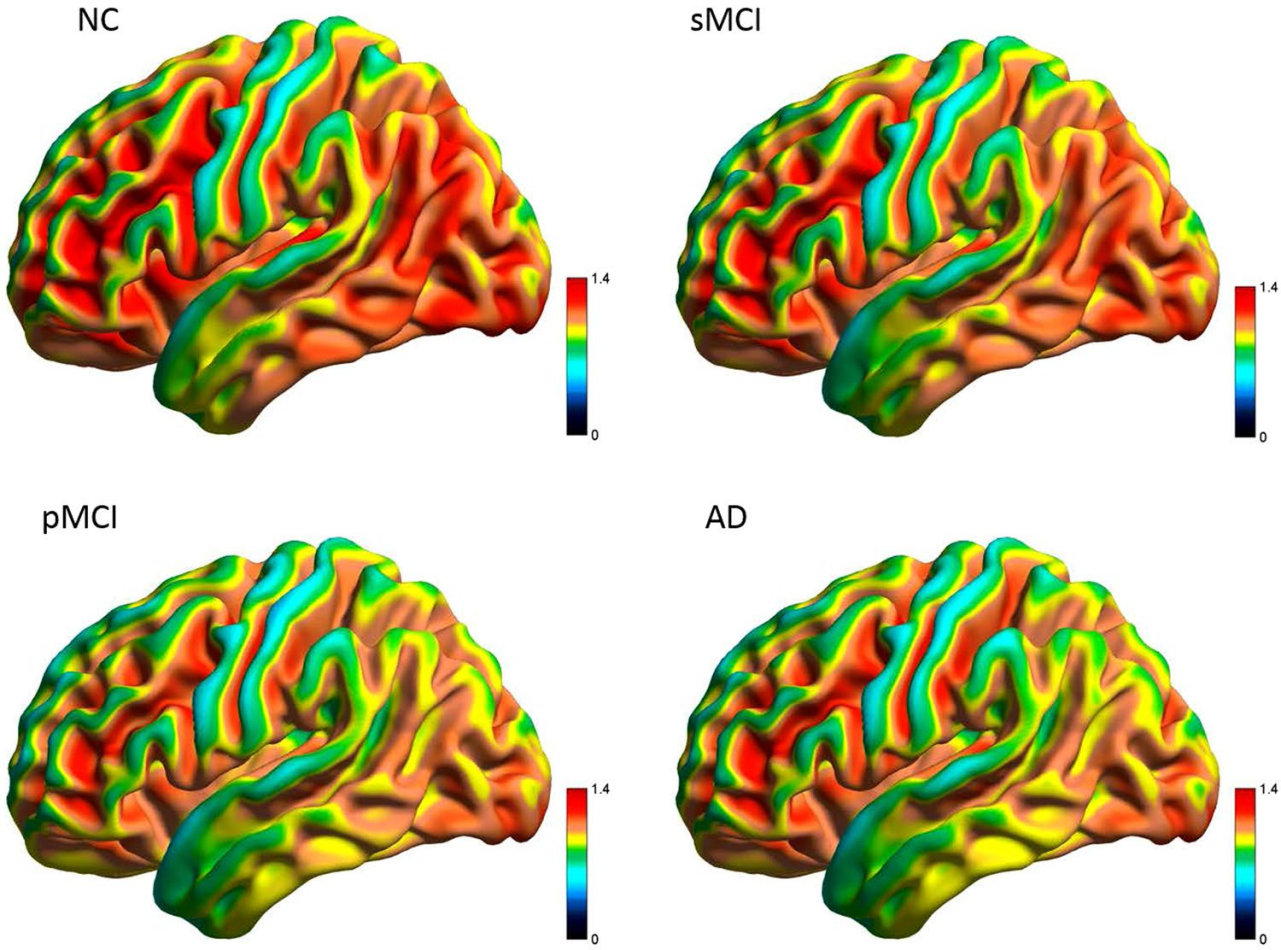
Three-dimensional visualization of mean SUVR uptake of FDG-PET in NC, sMCI, pMCI and AD from a lateral view.
